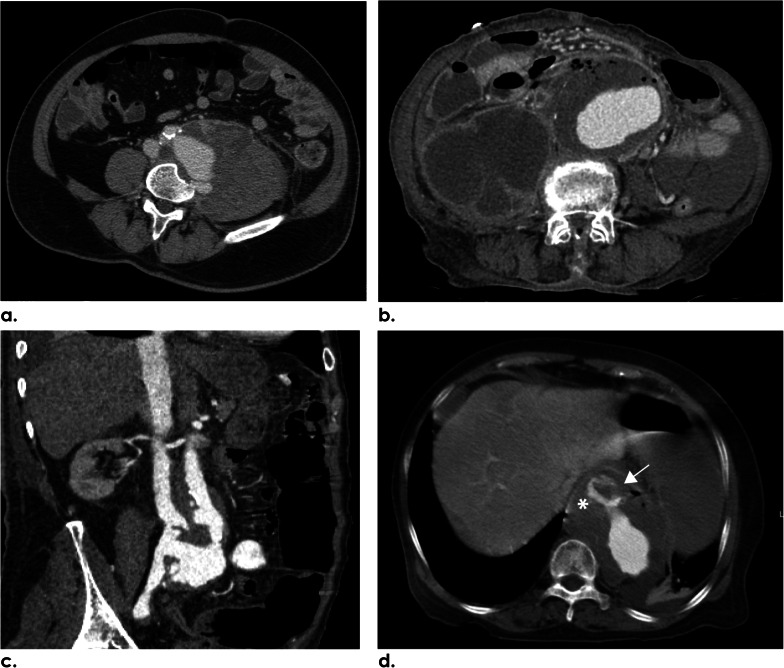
Surrounding organ involvement of INAA. a Axial CECT of a 58-year-old male patient depicts an infrarenal aneurysm with enhancing periaortic soft-tissue mass involving the left psoas muscle. The concave eroded anterior border of the adjacent vertebral body represents spondylitis. b Axial CECT of an 84-year-old female patient presenting with gastrointestinal hemorrhage reveals multiple air bubbles in the infrarenal aneurysm connecting to the adjacent duodenum, suggestive of aortoenteric fistula. A rim-enhancing fluid collection involving enlarged right psoas muscle is also noted. c Coronal angiographic CT image of a 66-year-old male patient reveals early enhancement of the IVC connecting to the aorta via multilobulated aortoiliac aneurysm, suggestive of aortocaval fistula. d Axial CECT of an 84-year-old female patient reveals active contrast extravasation (white arrow) from INAA into the esophagus. Asterisk (*) indicates aortoesophageal fistula. *INAA* infective native aortic aneurysm, *CECT* contrast-enhanced computer tomography, *IVC* inferior vena cava
